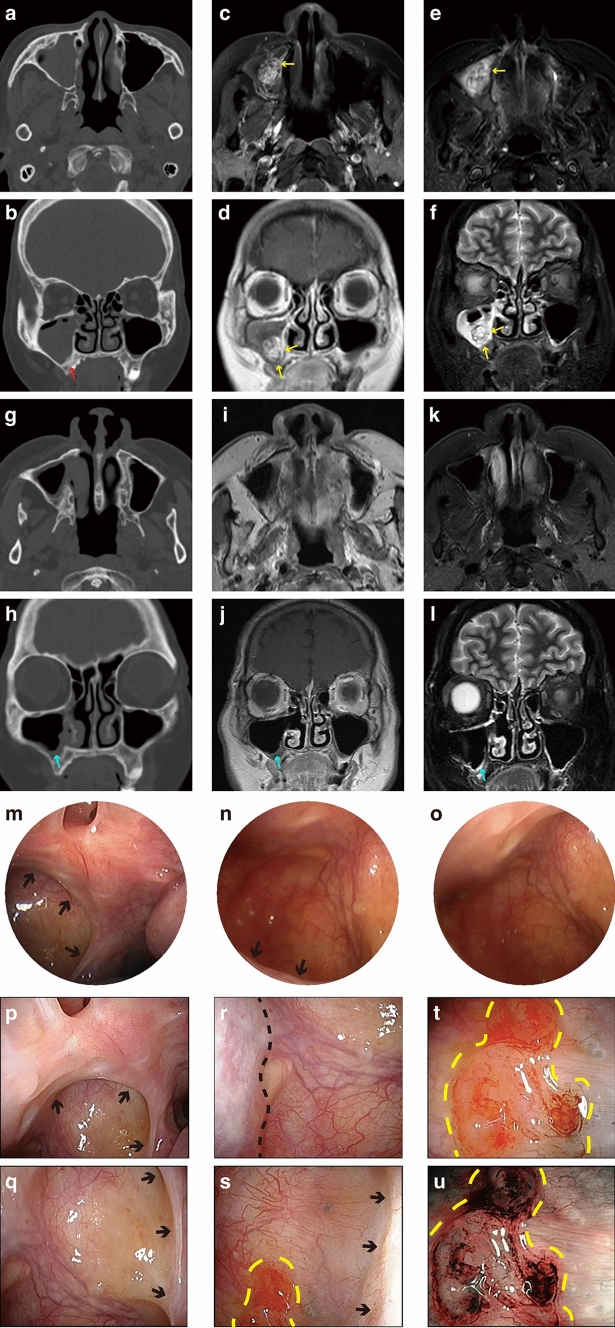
Lesion on the floor of MS detected by flexible endoscope in Case 2. a–f Preoperative medical images of the patient, including CT images (a, b), T1 weighted contrast-enhanced MRI (c, d) and T2 weighted MRI (e, f). The right MS with bone cortical discontinuity (red arrow) of the inferior wall was filled with lesions. The tumor located mainly at the medial and inferior wall (yellow arrows). g–l Images between two surgeries, including CT images (g, h), T1 weighted contrast-enhanced MRI (i, j) and T2 weighted MRI (k, l). The MS exhibited postoperative signals, suggesting thickening of the sinonasal mucosa and fibrotic scar probably (blue arrow). m–o Views of MS were observed by 0° (m) and 70° (n–o) endoscopes before second surgery. The lesion could hardly be found under 70° endoscope. p–u Views of MS were observed by flexible endoscope before second surgery. The anterior wall was presented (black dotted line). After entering the ostium (black arrows) of MS, the whole lesion (yellow dotted line) could be seen. The lesion was vascular dilatation under NBI module (u) (Color figure online)
